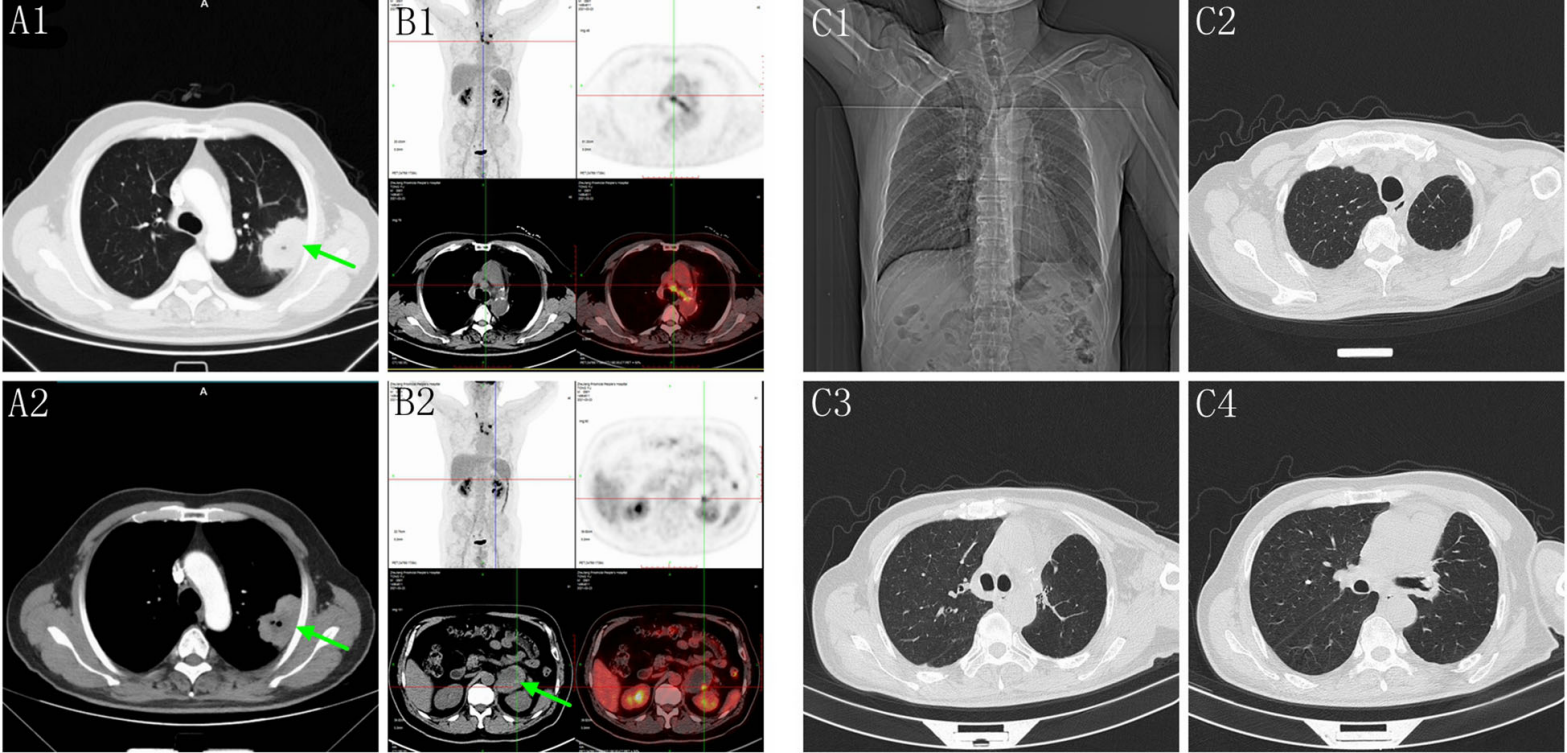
(A1, A2) Chest CT showed a solid mass in the superior lobe of left lung (green arrow). (B1, B2) Five months post-surgery, PET-CT revealed mediastinal adenopathy and a circular mass in the left adrenal gland measuring 8.5x5.6x4.3 cm (green arrow). (C1–C4) Chest CT showed no recurrence and the mediastinal lymph nodes were significantly reduced on September 26, 2024.
